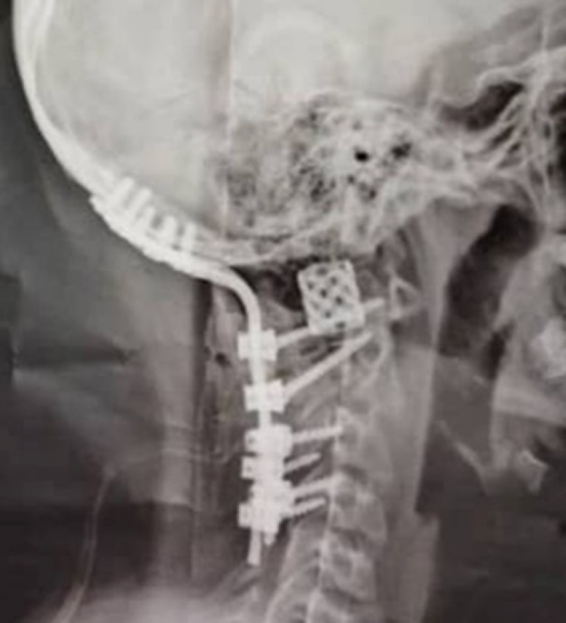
The cervical X-ray (lateral view) shows resection of the right C1 lateral mass ABC and reconstruction with a titanium mesh cage. Pedicle screws were inserted in the left side of the C1 and right side of C2, and the C3,4 vertebra was instrumented with lateral mass bilaterally. Finally, the patient underwent occipitocervical fusion from the occipital bone to the C4 vertebrae to reach craniocervical stability.
